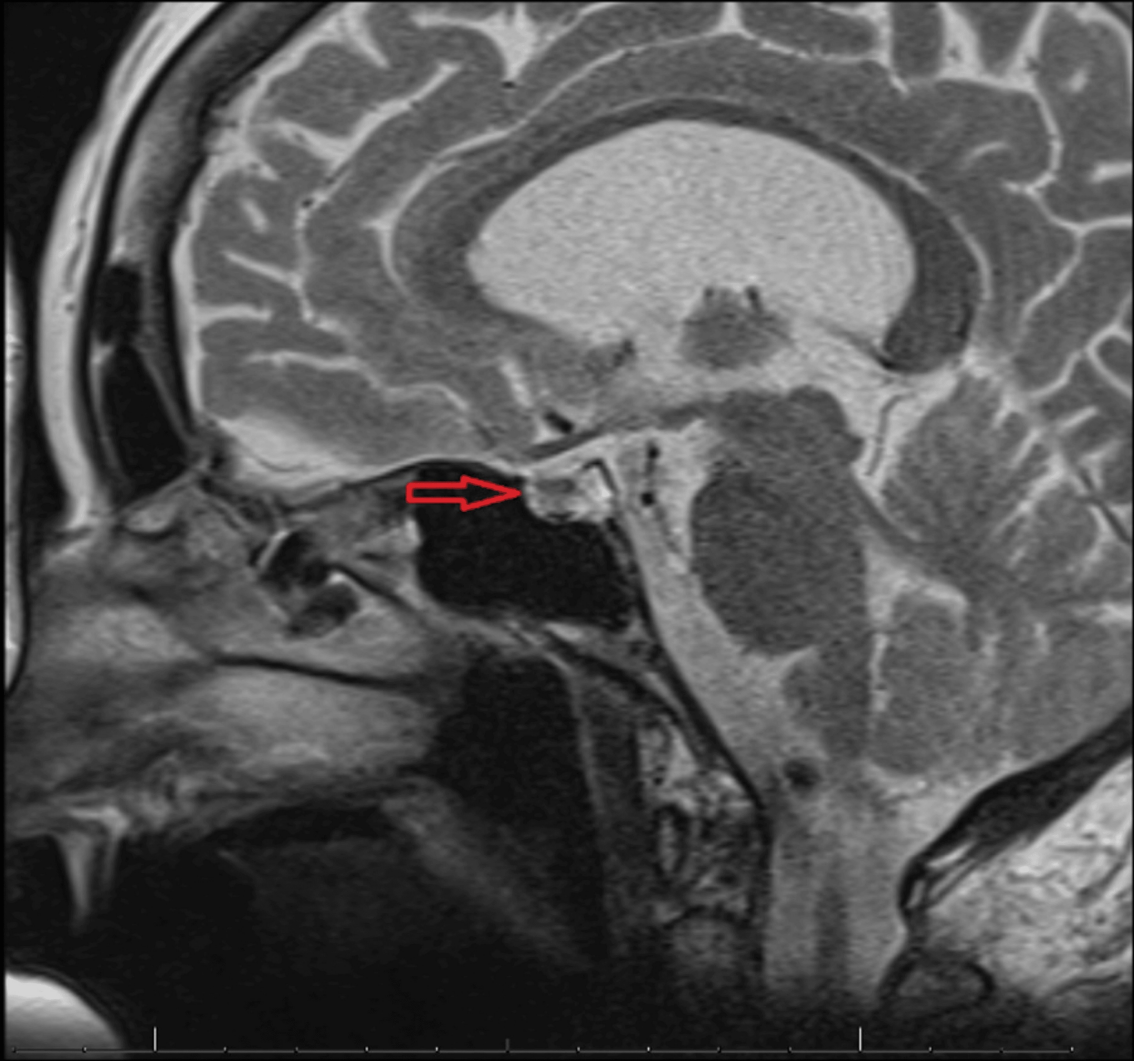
Normal noncontrast MRI of the sella
